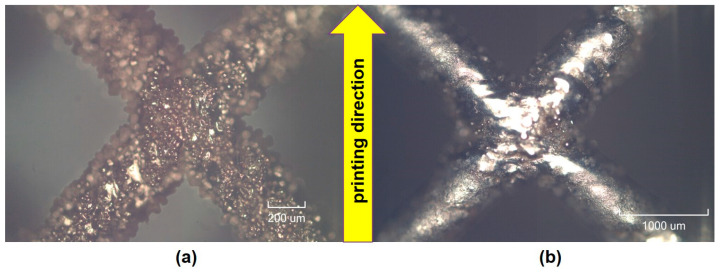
Stent struts (a) with spatter particles; (b) after ultrasonic tumbling.
